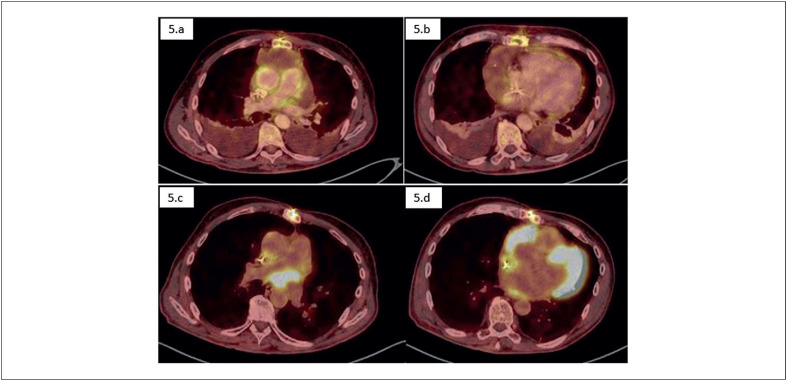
Imagens em cortes axiais de PET-SCAN usando o radiofármaco FDG que representam respectivamente: 5.a e 5.b) ausência de captações anômalas em 08/06/2023. 5.c e 5.d) captação anômala de radiofármaco evidenciando lesão expansiva hipermetabólica no leito cirúrgico atrial direito, em íntimo contato e sem planos de clivagem bem definidos com a parede lateral e posterior, além de surgimento de concentração do radiofármaco na topografia da base cardíaca em 03/01/2024.
